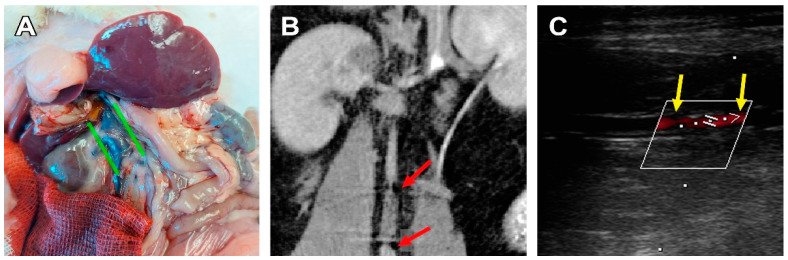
A case of graft stenosis 180 days after implantation. (A) Post-mortem examination, green lines indicate the caudal vena cava dilatation. (B) CBCT, coronal section, the red arrows indicate areas of insufficient contrast flow at the graft/vessel anastomoses. (C) An ultrasonic image of a stenotic graft, frontal section, the yellow arrows indicate the margins of the graft, and the red shadow indicates an area of insufficient blood flow.
